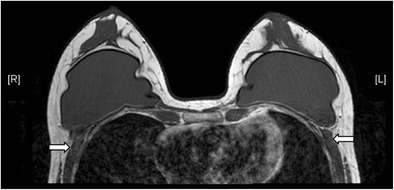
Retroglandular position of implants. T1-weighted axial MR image shows the retroglandular position of bilateral silicone gel-filled implants, which are entirely anterior to the pectoral muscles (arrows)
